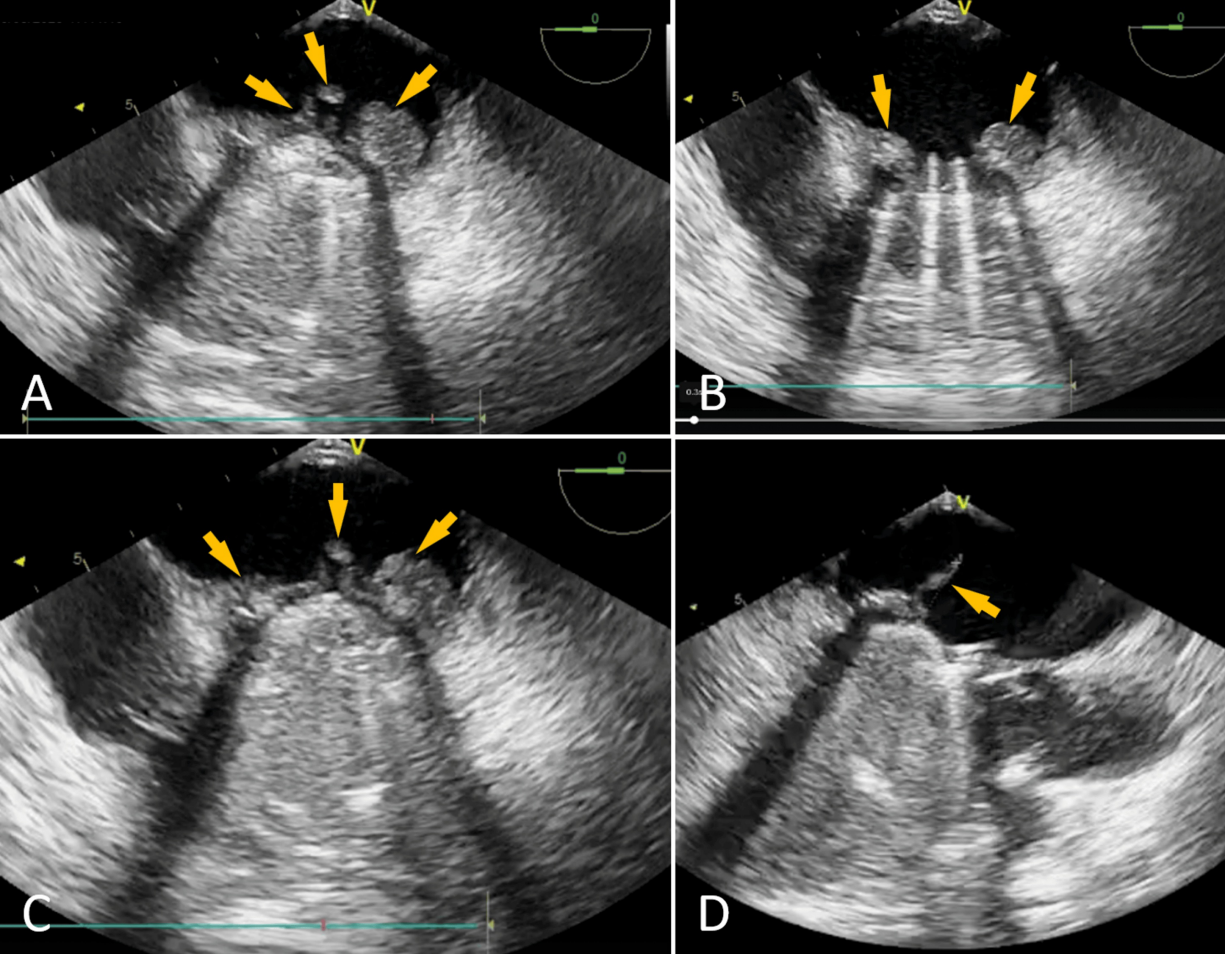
A, B, C: TEE four-chamber view, mid-esophageal: Large vegetation on the atrial side of the mechanical mitral prosthesis (Arrows). D: TEE cardiac long-axis view, mid-esophageal: Serpentine vegetation measuring 16 mm (Arrow) on the atrial side of the mechanical mitral prosthesis. TEE: Transesophageal Echocardiography
